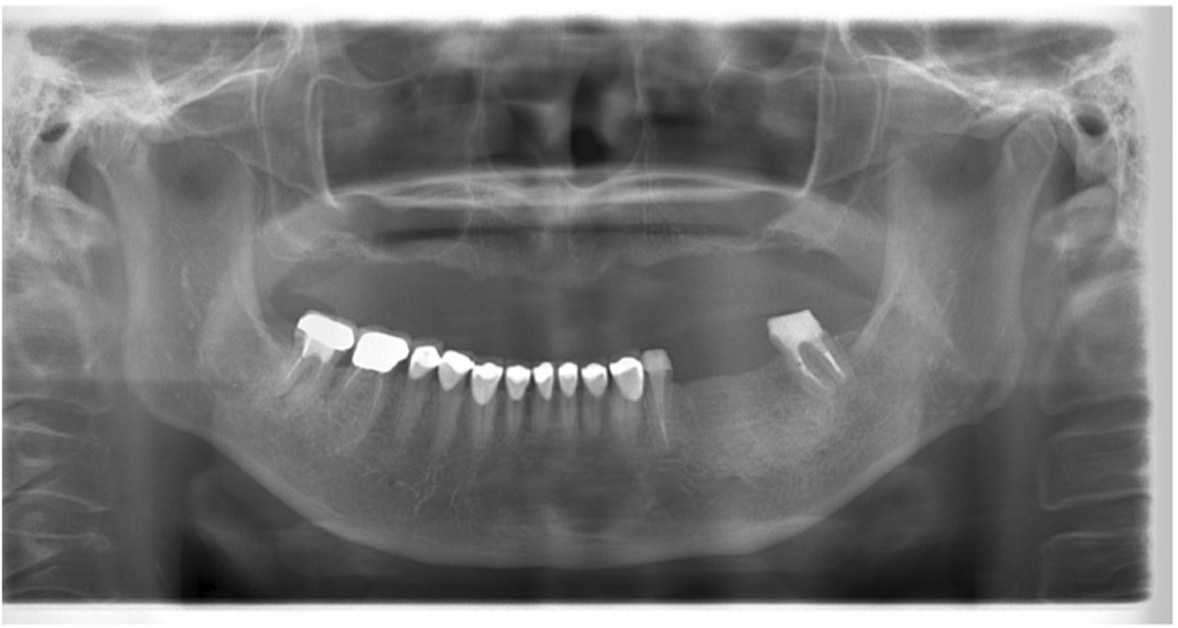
Panoramic radiography obtained before treatment.
